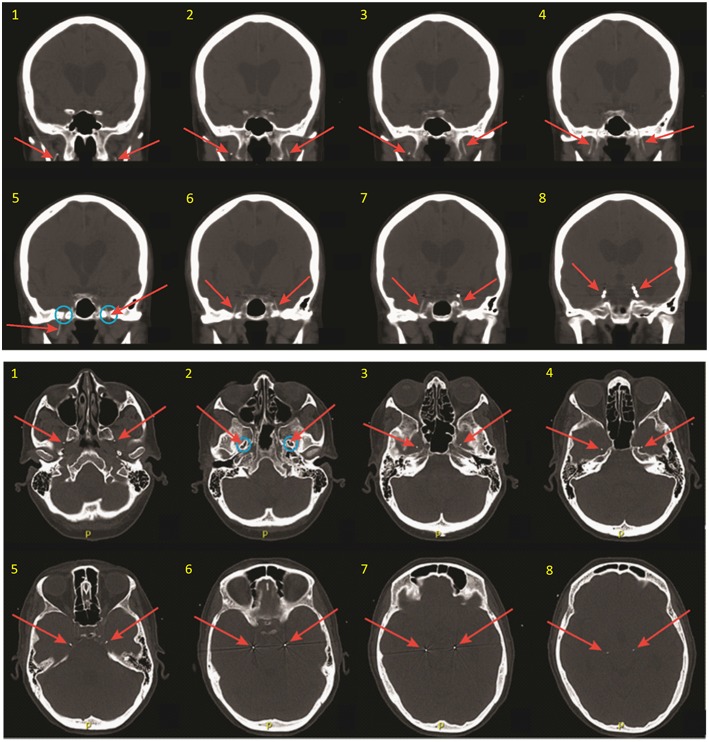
Coronal (top) and thin axial (bottom) views on post-operative CT scan, demonstrating FO electrode positioning. Slices are numbered in ascending order from anterior to posterior (coronal images), and from inferior to superior (axial images). Red arrows show the FO electrodes in each image. Note that the FO electrodes are only 1 mm in diameter but appear larger in these images due to image slice thickness and windowing needed for visualization. Blue circles show the foramen ovale.
